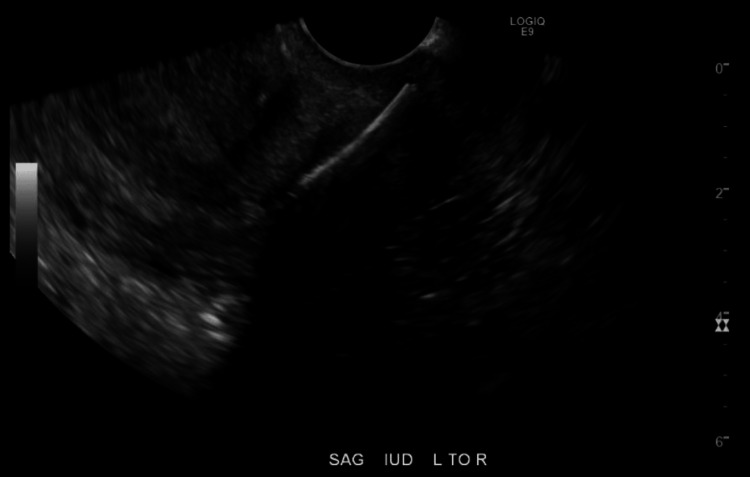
Endovaginal ultrasound in sagittal view with IUD fragment visualized in cervix with posterior acoustic shadowing. SAG: sagittal view; IUD: intrauterine device; L to R: left to right.
